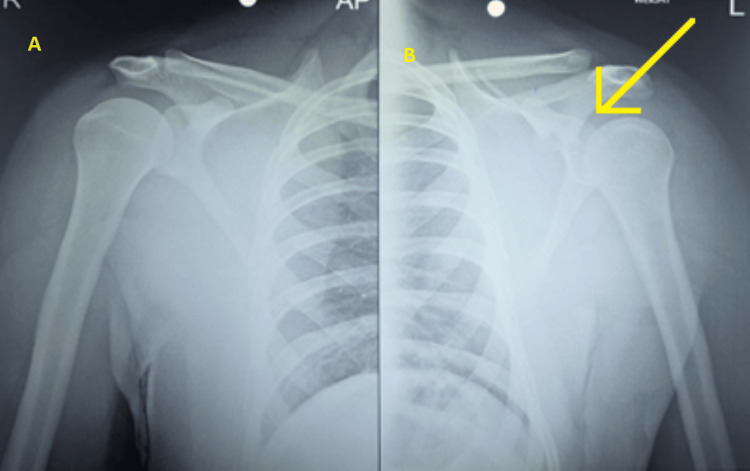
Plain radiograph at the time of presentation A) Right shoulder; B) Left shoulder The yellow arrow shows the fracture of the left acromion
